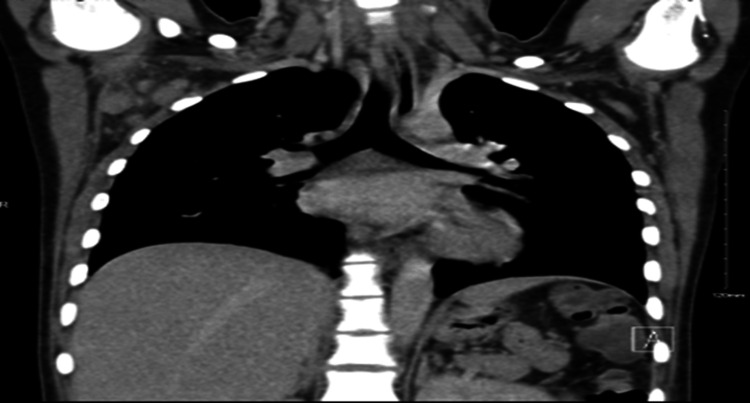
Whole body CT scan identified right axillary lymphadenopathy
